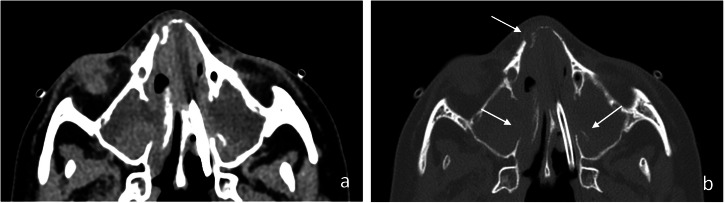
Proven invasive sinus mucormycosis in a 10-year-old girl after hematopoietic stem cell transplantation therapy. Axial CE-CT images of paranasal sinuses in soft tissue (a) and bone window (b) show bilateral complete opacification of the maxillary sinus with heterogeneous, partially hyperintense content. Note extensive bone erosion and lytic destruction of the nasal bones (arrows, b)
